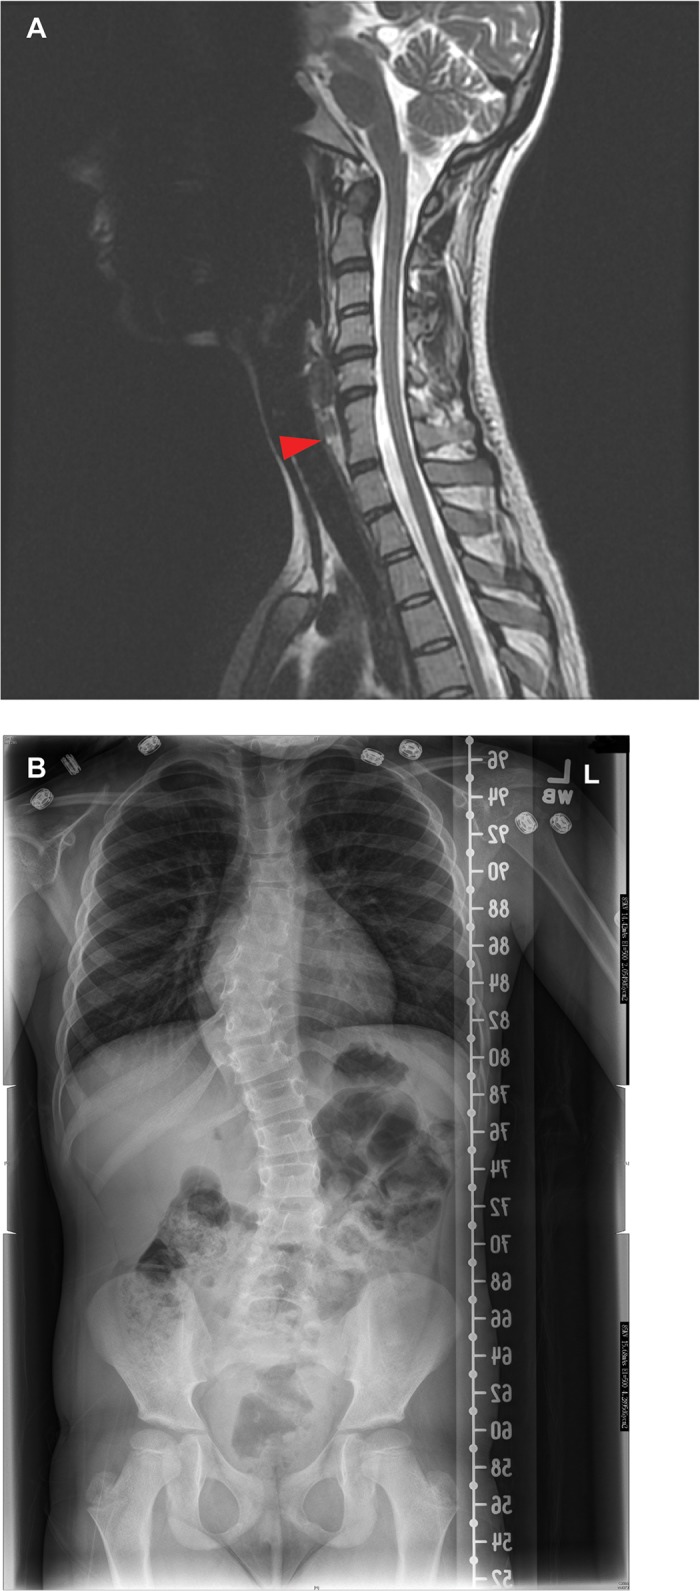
Spinal fusion anomalies in NF1 patients. (A) Congenital C6–7 vertebral fusion in a 24 year old, female patient with NF1. MRI shows congenital fusion of the 6th and 7^th^ thoracic vertebrae with an absent disc in a 24 year old woman with a clinical diagnosis of neurofibromatosis type 1 (NF1) based on her history of multiple cafe-au-lait spots, axillary freckling and dermal neurofibromas. (B) Segmental fusion anomalies at T8-T10 in a 7 year old female NF1 patient with dystrophic scoliosis. An anterior-posterior standing radiograph of a 7 year old girl with the clinical diagnosis of NF1 (i.e. >6 café au lait macules >0.5cm, axillary and groin freckling, and a first-degree relative with NF1) shows a right thoracic curve measuring 32 degrees between the 8th and 12th thoracic vertebrae and a left thoracolumbar curve measuring 23 degree between the 12th thoracic and 3rd lumbar vertebrae with segmentation and vertebral fusion anomalies at the 8th-10th thoracic vertebrae.
